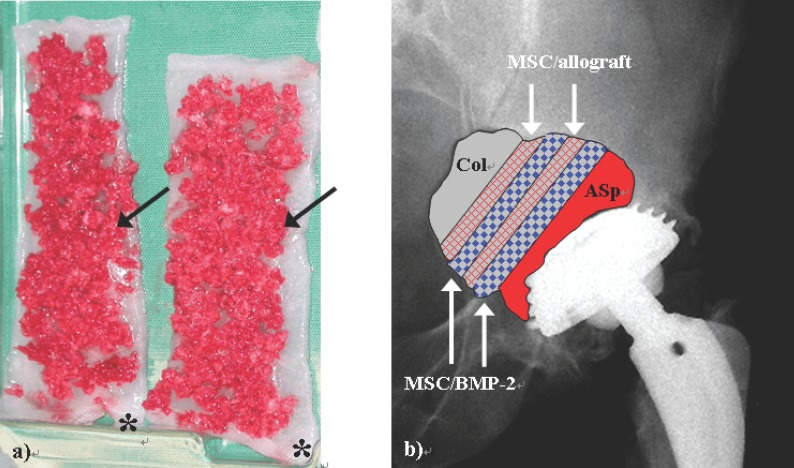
a) The figure shows two collagen I/III strips (*) which were impregnated by BMP-2, covered by MSCs containing autologous human bone marrow which was harvested by vacuum aspiration and augmented by spongiosa chips from iliac crest (arrows). b) The x-ray on a.p. view shows the multilayer sandwich-technique of refilling the critical bony osteolysis behind the cup. To lower the risk of an ectopic bone formation at the inner pelvic site collagen I/III sponges should act as a barrier and were positioned at the medical site of the reconstructive area (Col). Allograft bone was augmented by MSC containing bone marrow aspirate (MSC/allograft) and BMP-2 containing collagen I/III sponges were incubated with MSCs (MSC/BMP-2). Col: collagen I/III sponge, MSC(s): bone marrow derived mesenchymal stem cell(s), ASp: autologous spongiosa, BMP: bone morphogenic protein.
